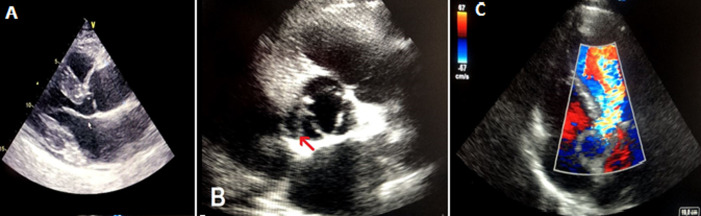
transthoracic echocardiography: (A) a parasternal long-axis view showing dilated aortic root at sinus of Valsalva, left ventricular hypertrophy and small pericardial effusion; (B) a parasternal short-axis view of the aortic valve showing the dissection flap (arrow); (C) apical 5-chamber view of the severe aortic regurgitation in color doppler study
